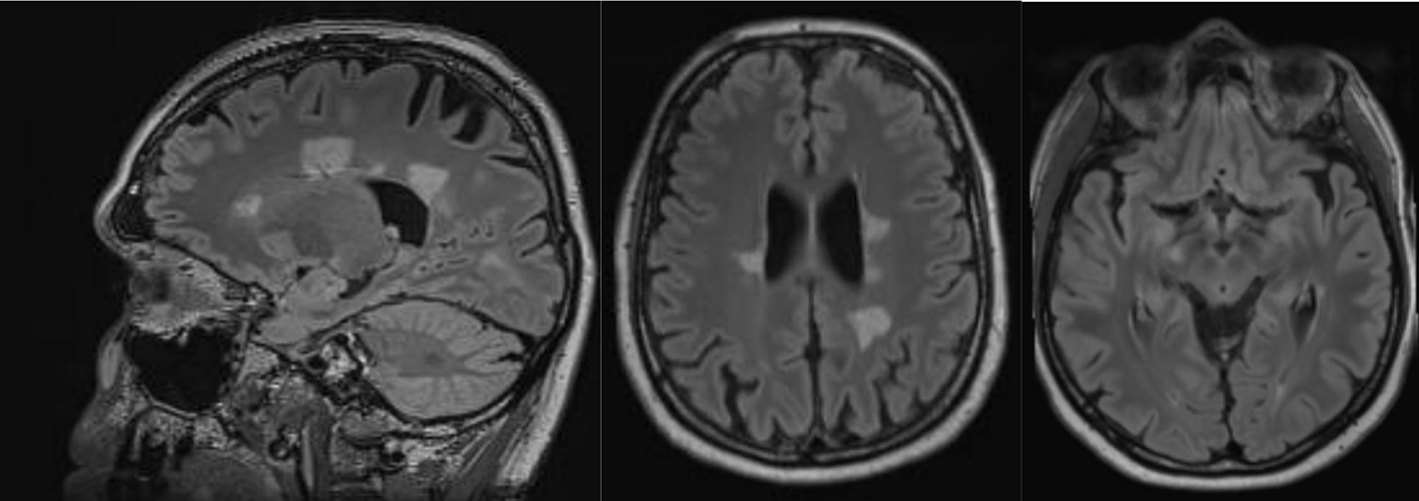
Magnetic resonance imaging showed conspicuous supratentorial white matter lesions without contrast enhancement. This is compatible with chronic inflammatory brain disease.
